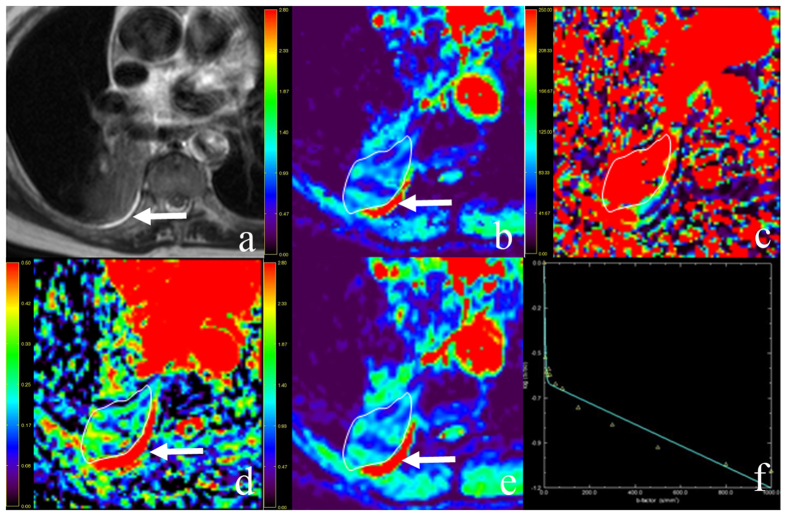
Representative imaging characteristics in a 65-year-old man with right lower lobe adenocarcinoma. (a) T2-weighted image. (b) The D map. (c) The D* map. (d) The f map. (e) Apparent diffusion coefficient (ADC) map. (f) The bi-exponential fitting of the diffusion signal decay. The tumor demonstrates slightly high signal on T2WI and mixed color on D and ADC maps. Dispersed blue and violet areas (representing regional restricted diffusion) were observed, which indicates a malignant tumor. The f map demonstrates a relatively high perfusion fraction region compared to the lung. The D* map shows a high pseudo-diffusion values in the tumor tissue. Pleural effusion (arrow) demonstrates as hyperintensity on T2WI as well as high value on D, ADC and f maps (encoded in a red color).
